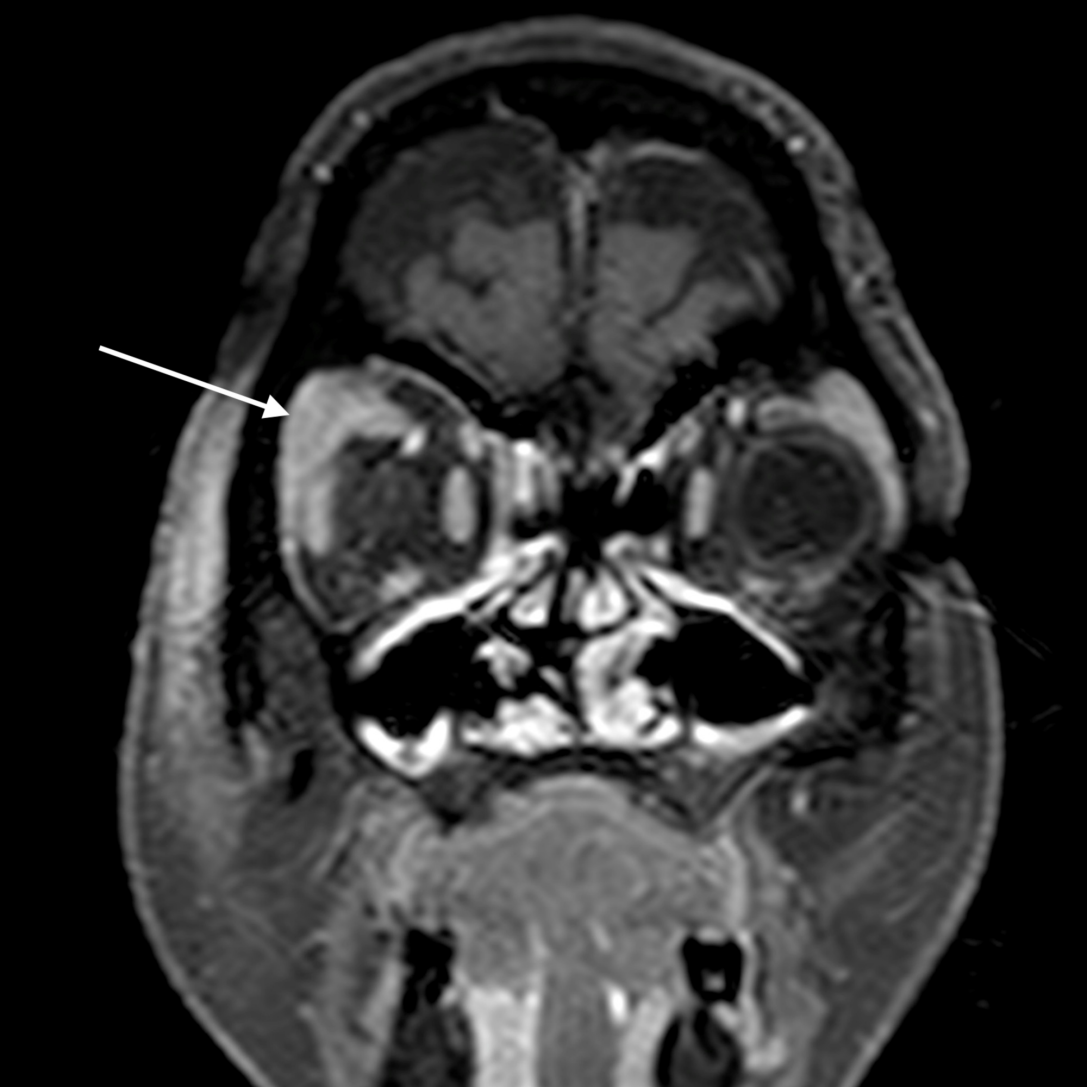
Dacryoadenitis: coronal post gadolinium T1WI shows abnormal enhancement of the enlarged lacrimal gland (arrow) T1WI - T1-weighted image
